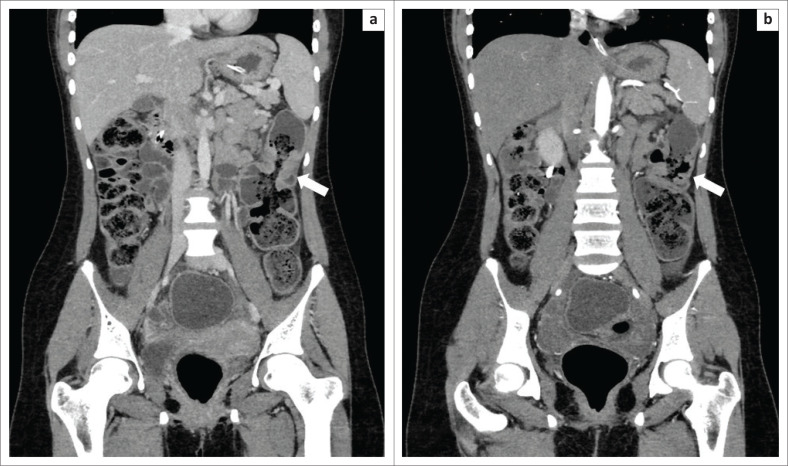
(a and b) Coronal reformatted computed tomography images of a 19-year-old female with a history of repeatedly chewing her ‘dupatta’ (a shawl-like scarf traditionally worn by women of the Indian subcontinent) showing a short segment of bowel wall thickening in the proximal descending colon (arrows). The ‘faecal like’ material within the lumen of the affected descending colon was identified at laparoscopy as textile bezoar.
